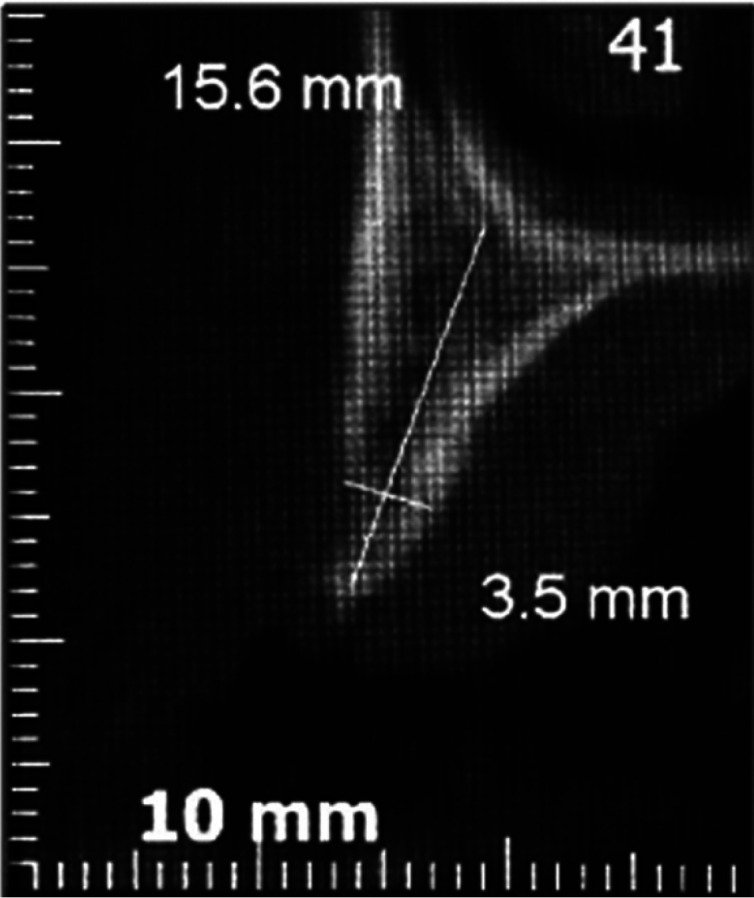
Six-month post-surgery cone beam computed tomography scan showing bone neoformation in the area.
